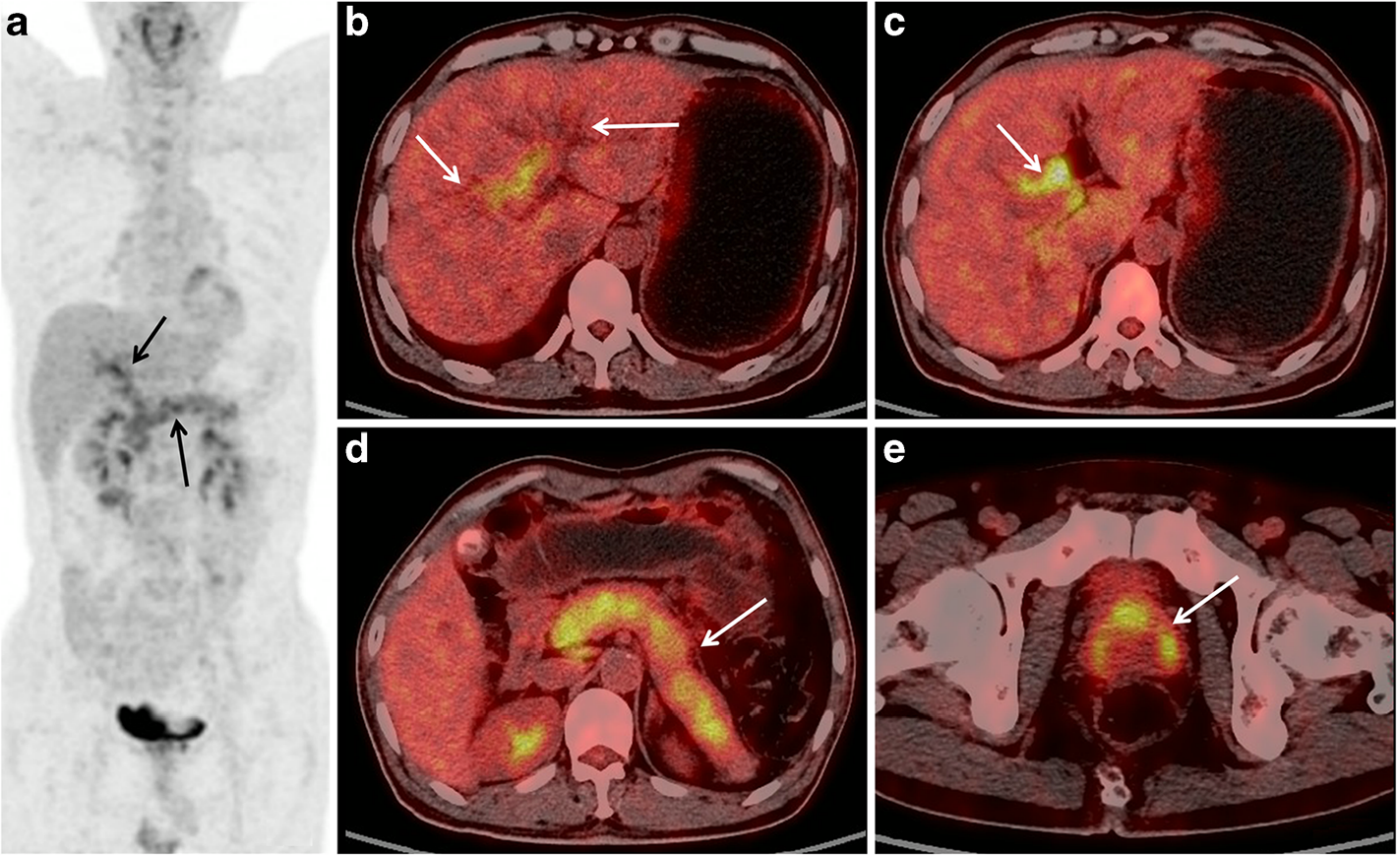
A 55-years old male patient with AIP. The MIP PET image (a) shows a diffuse and heterogeneous increase of FDG uptake in the pancreas, as well as increased FDG activity along the bile duct; PET/CT fusion images (b) depicts bile duct dilatation; (c), increased FDG activity of the hilar bile duct is shown; (d) shows diffusely enlarged pancreas with capsule-liked rim and a heterogeneous increase of FDG uptake. e, there is an inverted “V” shaped high FDG uptake in the prostate
